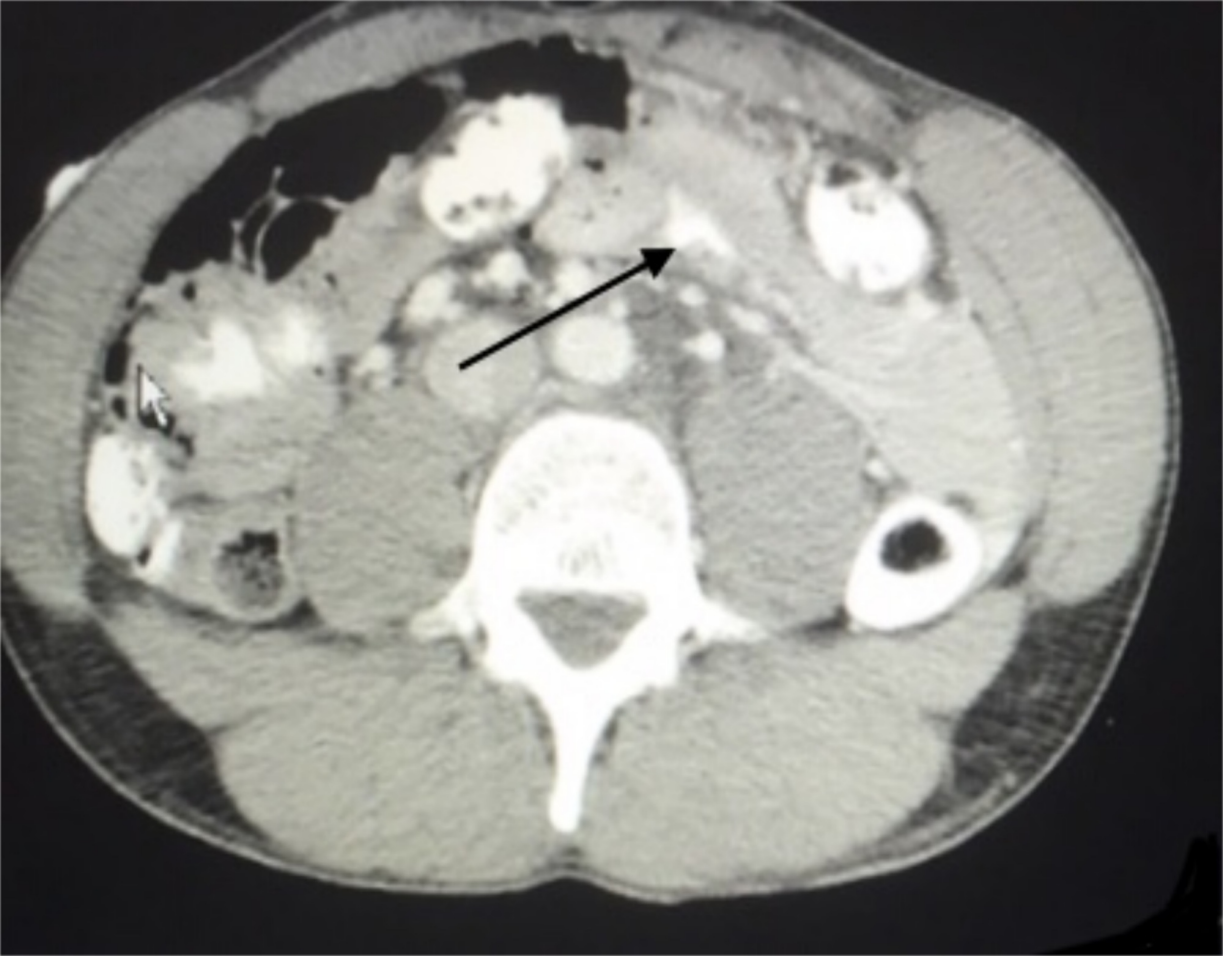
CT scan abdomen Oral contrast extravasation (black arrow) CT: computed tomography
